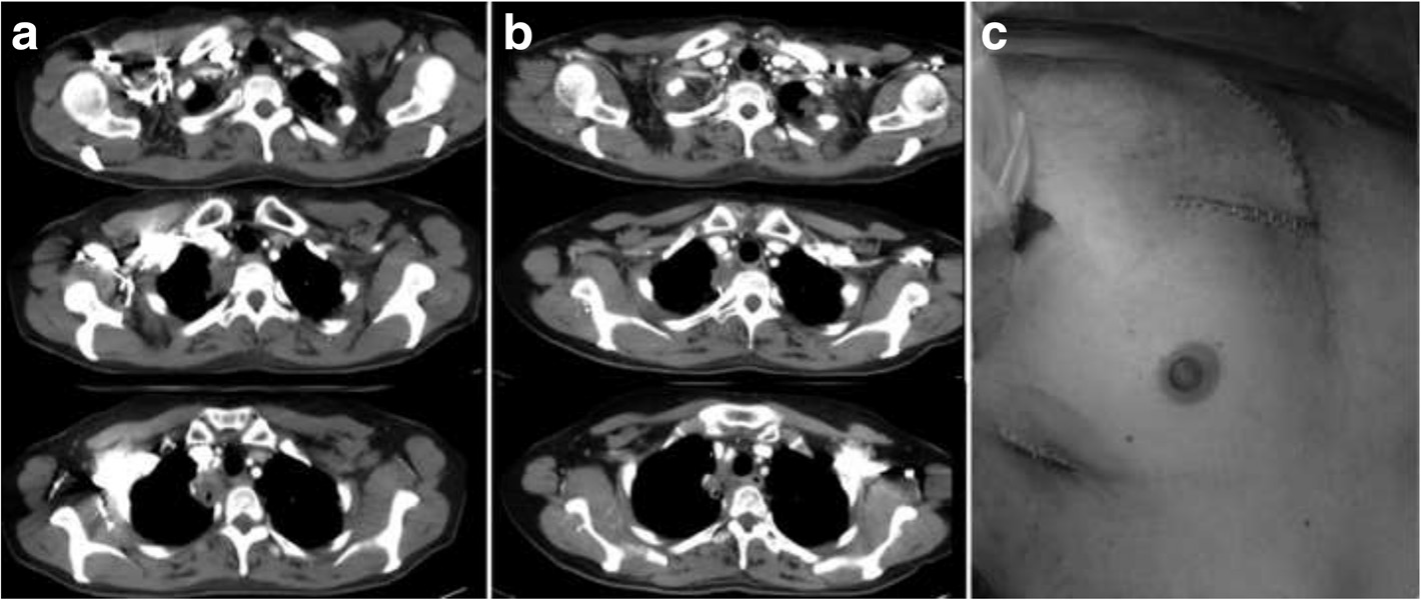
a Pre-induction therapy CT scan; b Post-induction therapy CT scan; c Modified Grunenwald incision and VATS incision
